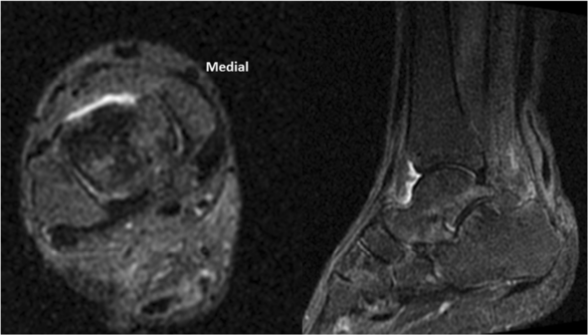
MR images obtained 58 days after surgery. Disappearance of tendon gap appears in T2 with fat sat.
